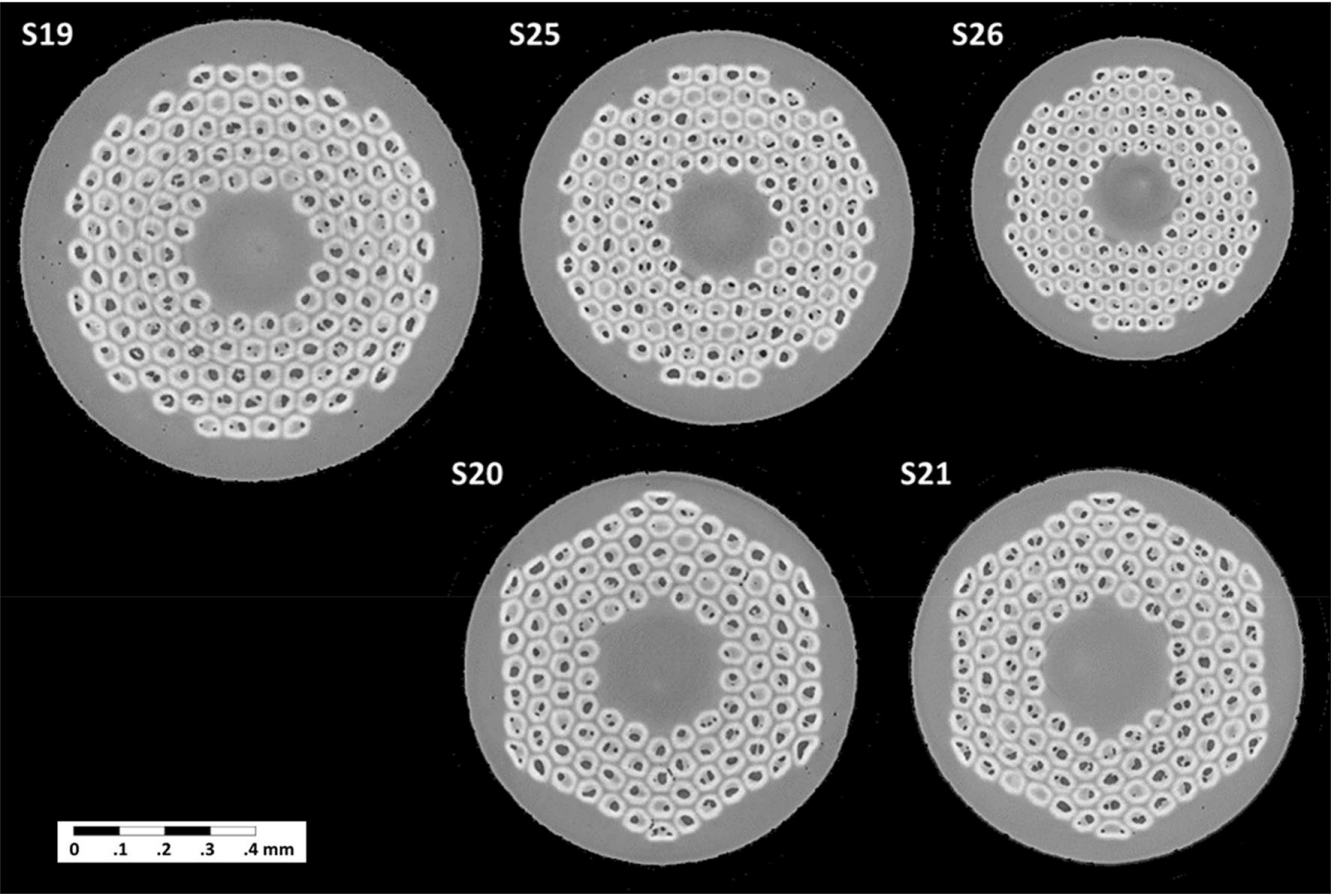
X-ray tomography cross section of the Nb_3_Sn RRP wires tested at the ESRF facility.
